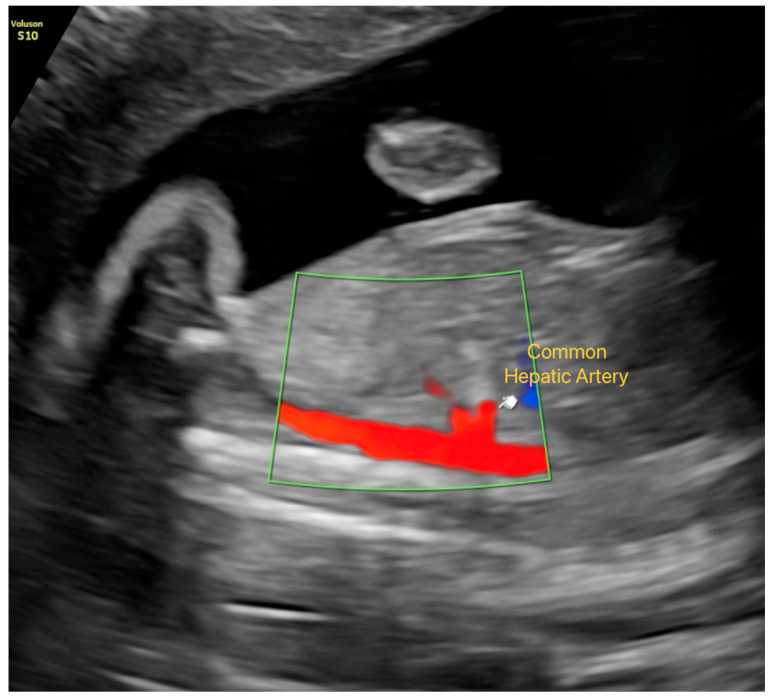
Visualization of the Common Hepatic Artery in Late-Gestation Fetal Ultrasound. Color Doppler imaging showing the common hepatic artery in a sagittal plane immediately distal to its origin from the celiac trunk.
